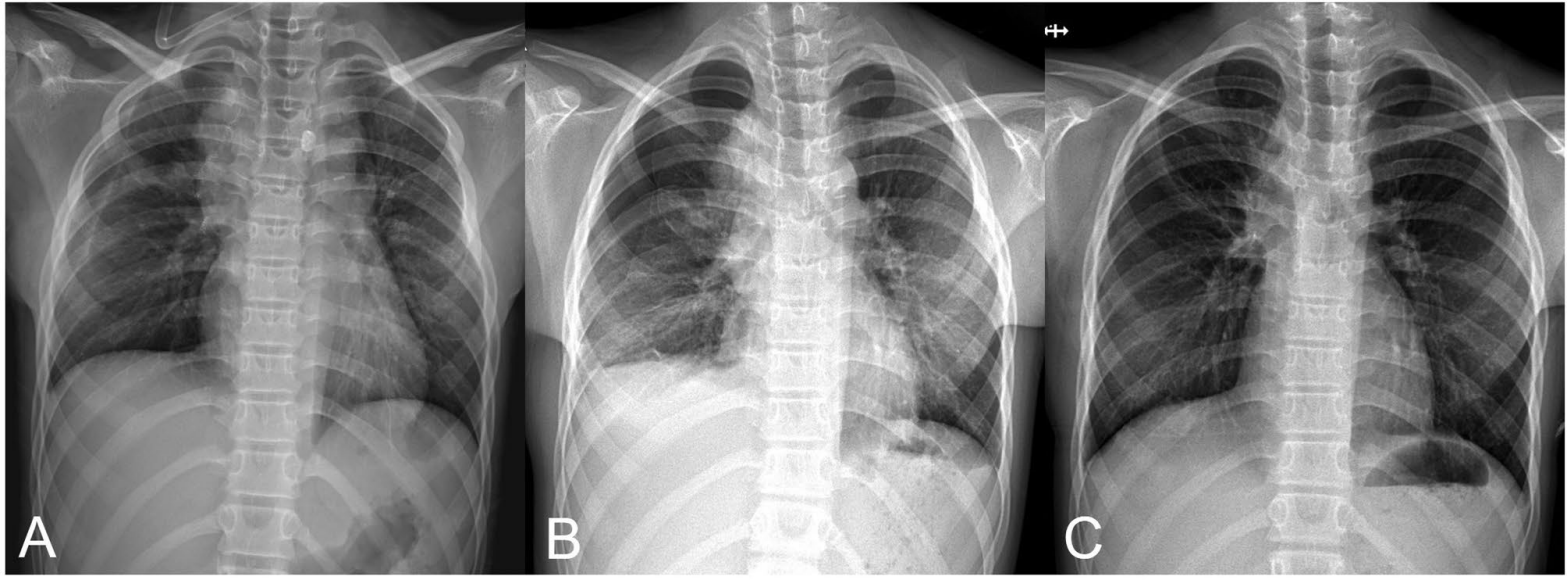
Postoperative chest radiographs: (A) clear upright anteroposterior film acquired in recovery room; (B) residual pneumothorax detected on postoperative Day 2; and (C) spontaneous resolution confirmed at first outpatient visit
